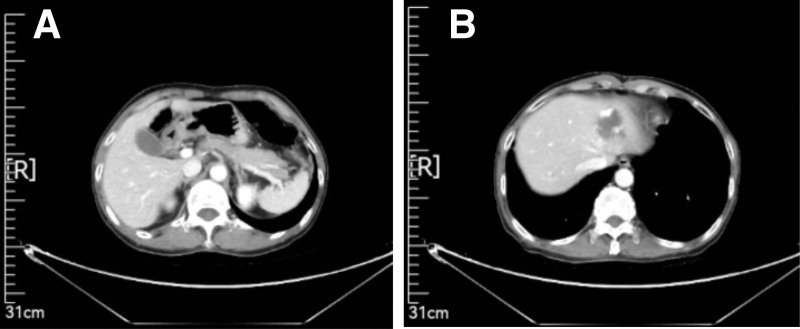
CT (April 26, 2020) revealed a slightly thickened gastric antrum and a left lobe hemangioma of the liver.
